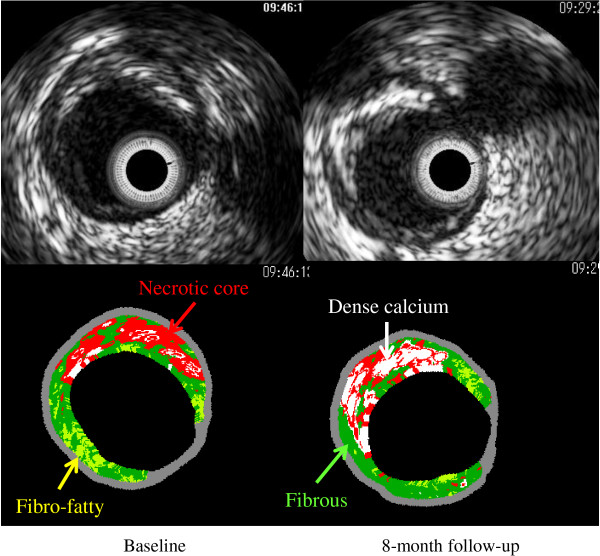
Representative intravascular ultrasound images of necrotic core regression. This case was a 78-year-old male patient with stable angina pectoris treated with pravastatin. His baseline serum lipoprotein(a) level was 3 mg/dL. A greater reduction of the necrotic core area was observed at the 8-month follow-up.
